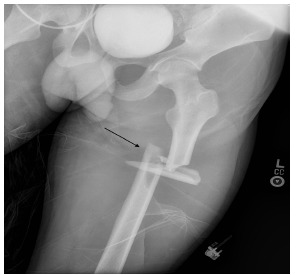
Radiograph of the left hip demonstrating segmental, displaced, and shortened left femur fracture (arrow).
